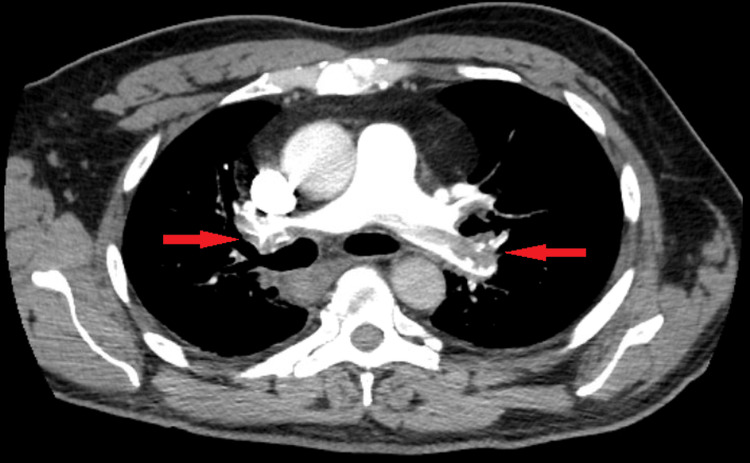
CT angiography of the chest showing bilateral filling defects (red arrows) in the main pulmonary arteries and their tributaries.
